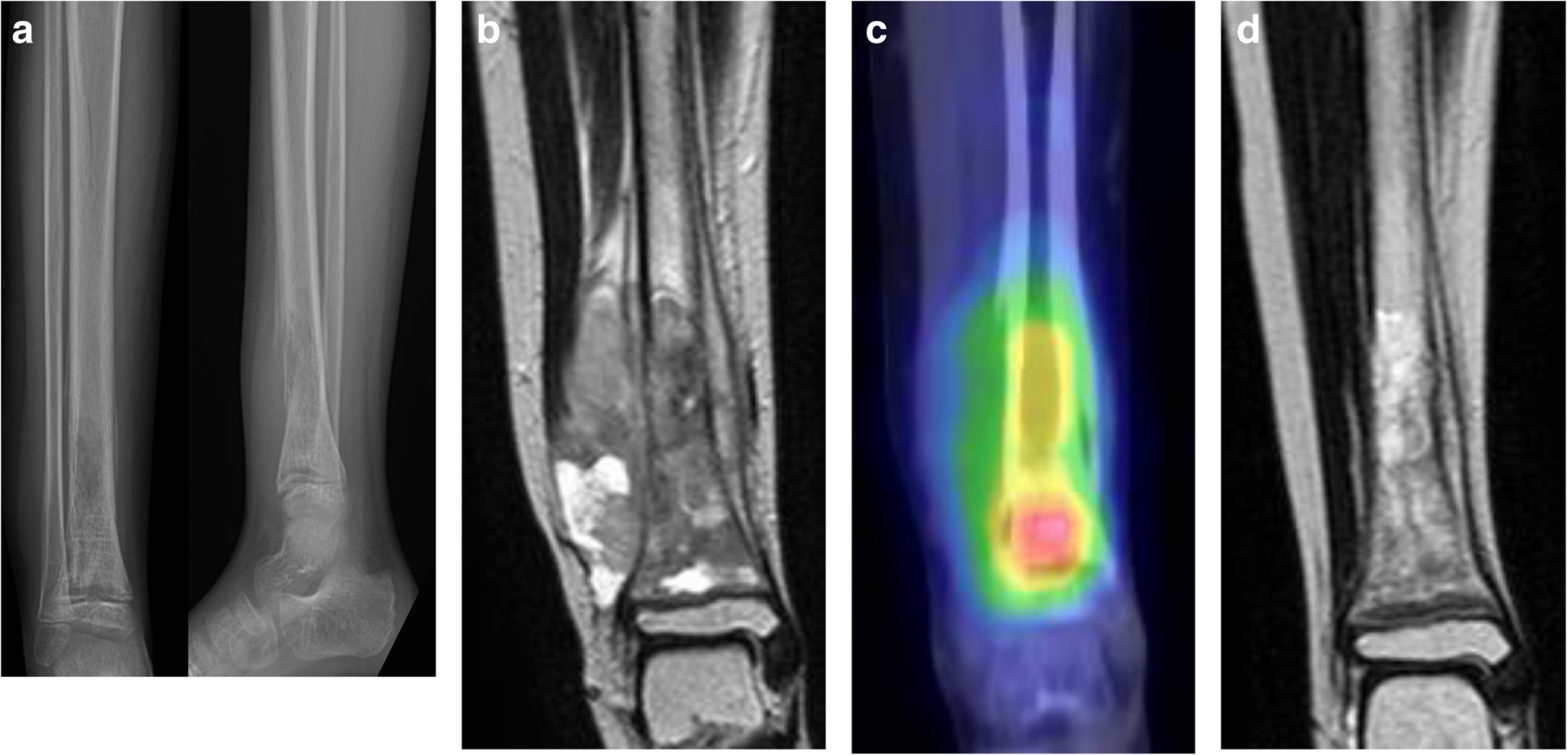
a X-ray of the lower leg showing periosteal response, and translucent distal tibia. b MRI coronal T2 weighted image showing massive extra-skeletal mass at the anterior distal metaphysis of tibia. c Thallium scan, showing high accumulation at the distal metaphysis of tibia. d MRI coronal T2 weighted image following chemotherapy revealing reduction in the extra-skeletal mass
